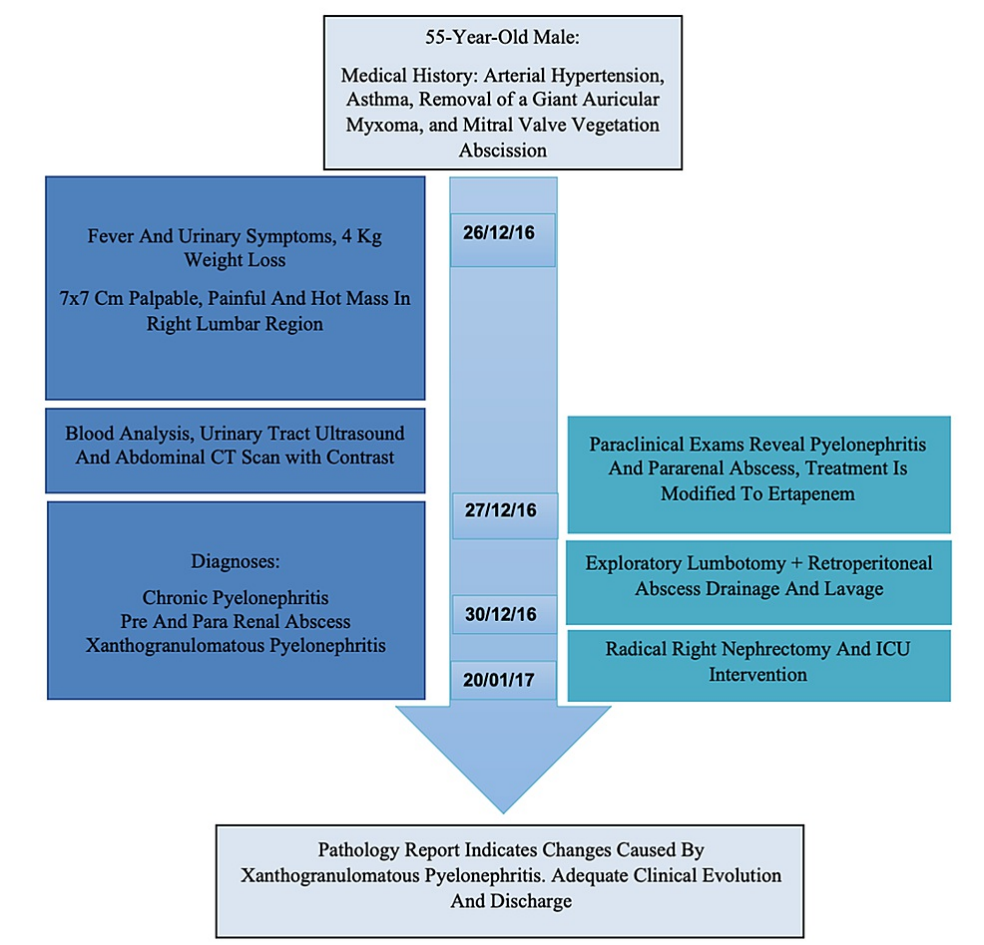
Timeline with significant dates CT: computed tomography; ICU: intensive care unit
